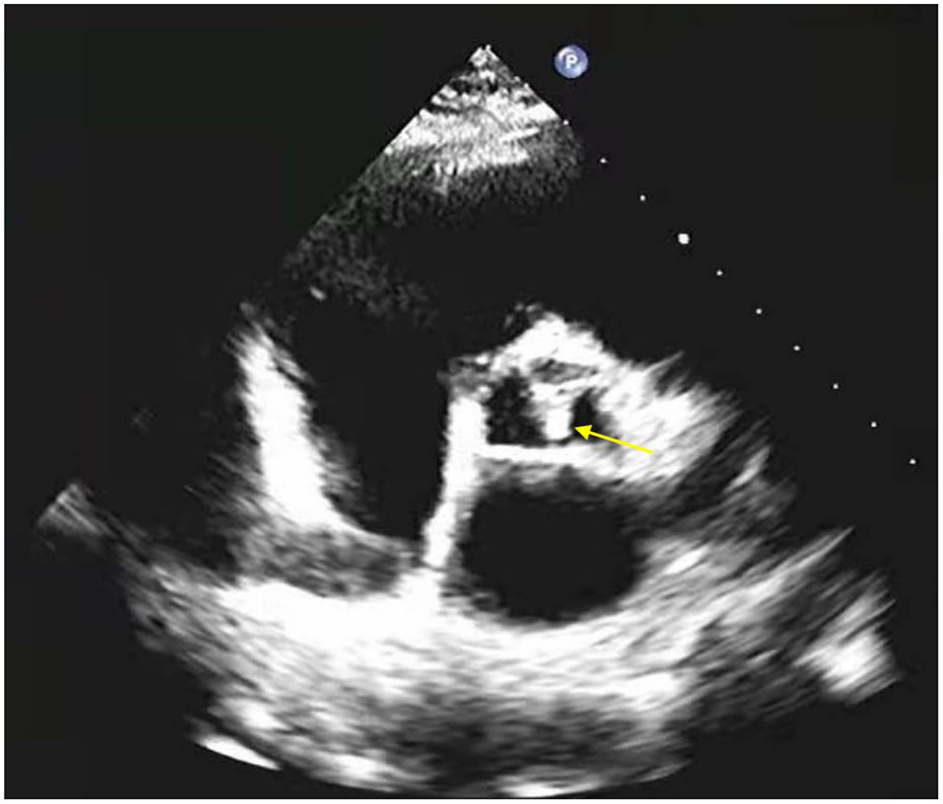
Example of echocardiogram showing calcification of the aortic valve (yellow arrow).
